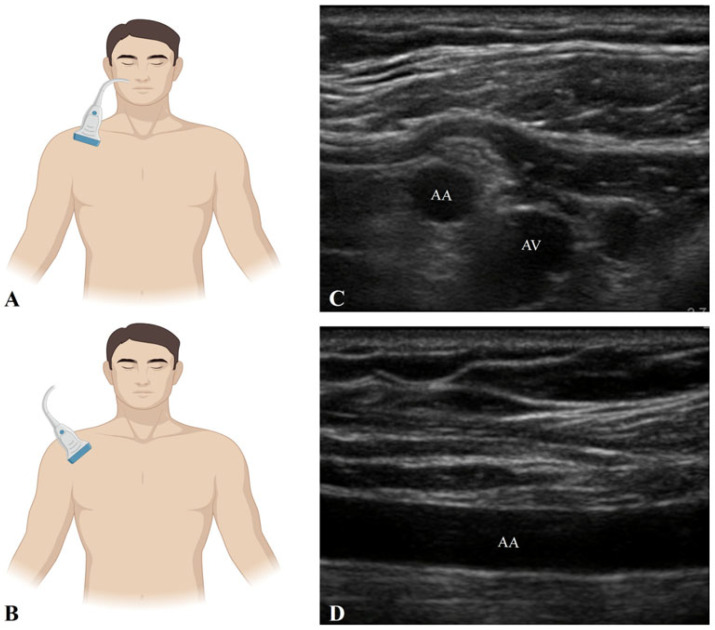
Sonographic assessment of the axillary artery. (A) Linear probe positioned transversely, perpendicular to the axis of the upper limb. (B) Linear probe positioned longitudinally, parallel to the axis of the upper limb. (C) Out-of-plane view of the axillary artery and axillary vein. (D) In-plane view of the axillary artery. AA: Axillary artery; AV: Axillary vein. Figure Author generated using BioRender 201.
